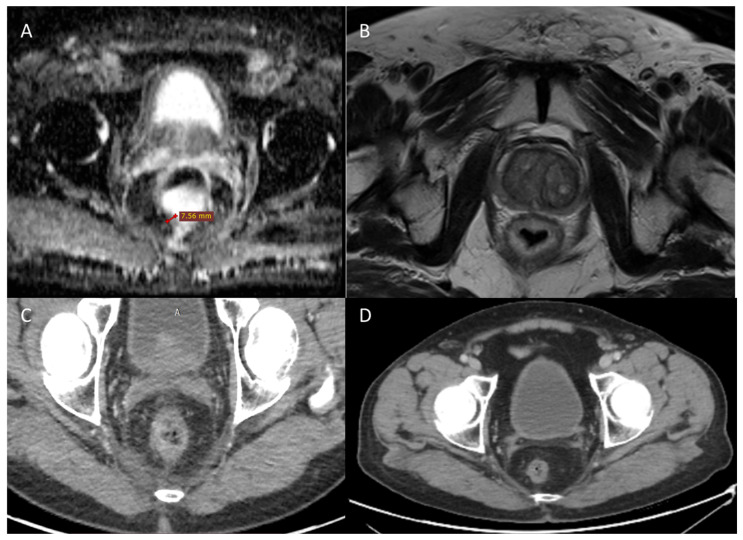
MR and CT images (A,B) before treatment of first case and CT images at 6 and 9 months after ECT treatment in (C,D), respectively.
